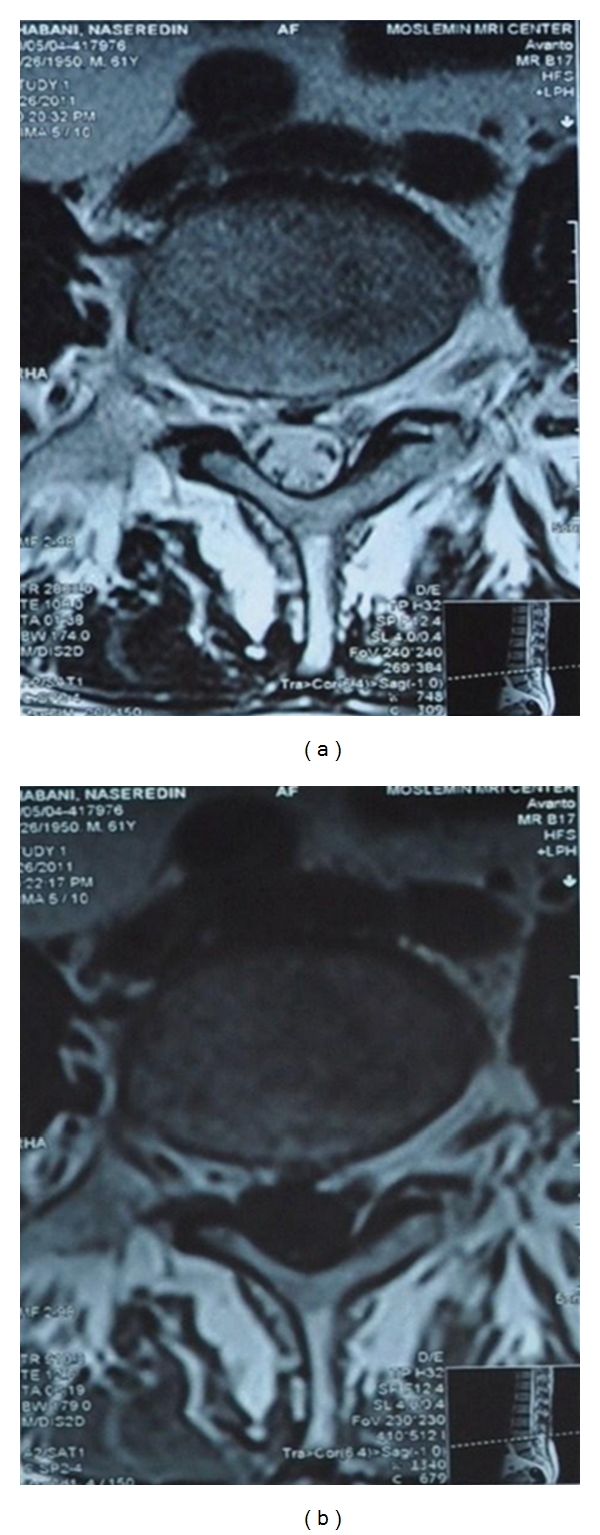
Axial T1- (a) and T2-weighted (b) lumbar MR images showed central protrusion of L4-L5 disc with slight nerve roots compression bilaterally.
